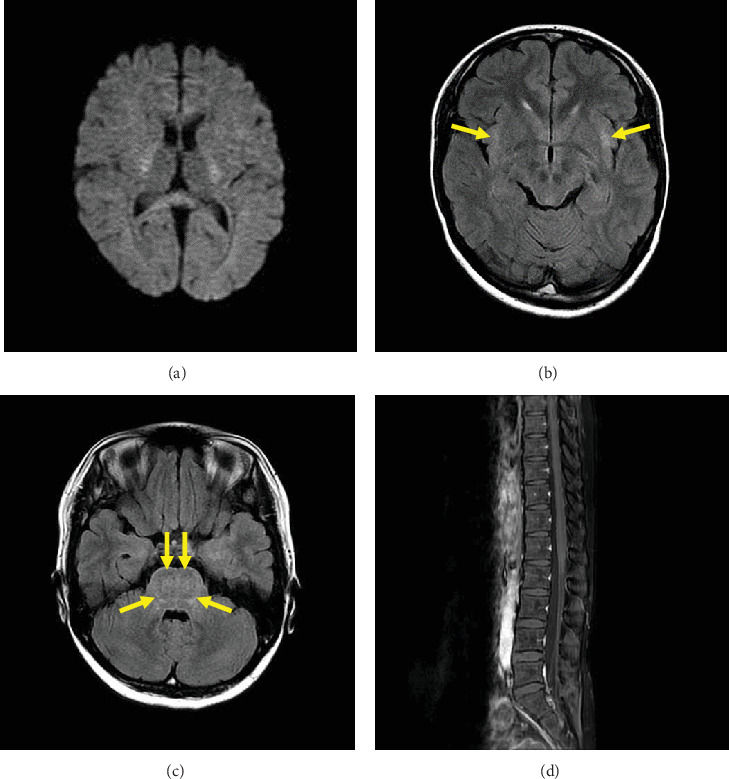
Magnetic resonance imaging (MRI) on Day 14. DWI showed reduced signal intensity in the SCC (a). FLAIR images revealed high signal intensity in the external capsule (b, arrows) and pons (c, arrows). Sagittal T1-weighted contrast-enhanced images of the lower spine showed no abnormal enhancement (d).
